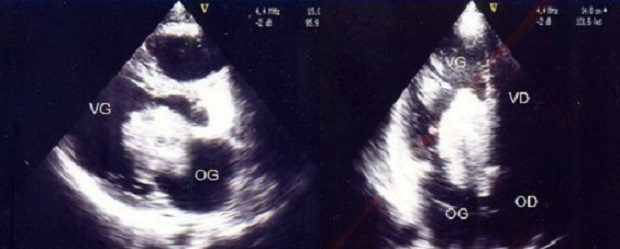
Echography showing the left atrial myxoma. At left: Parasternal long axis, at right: 4 chambers view
